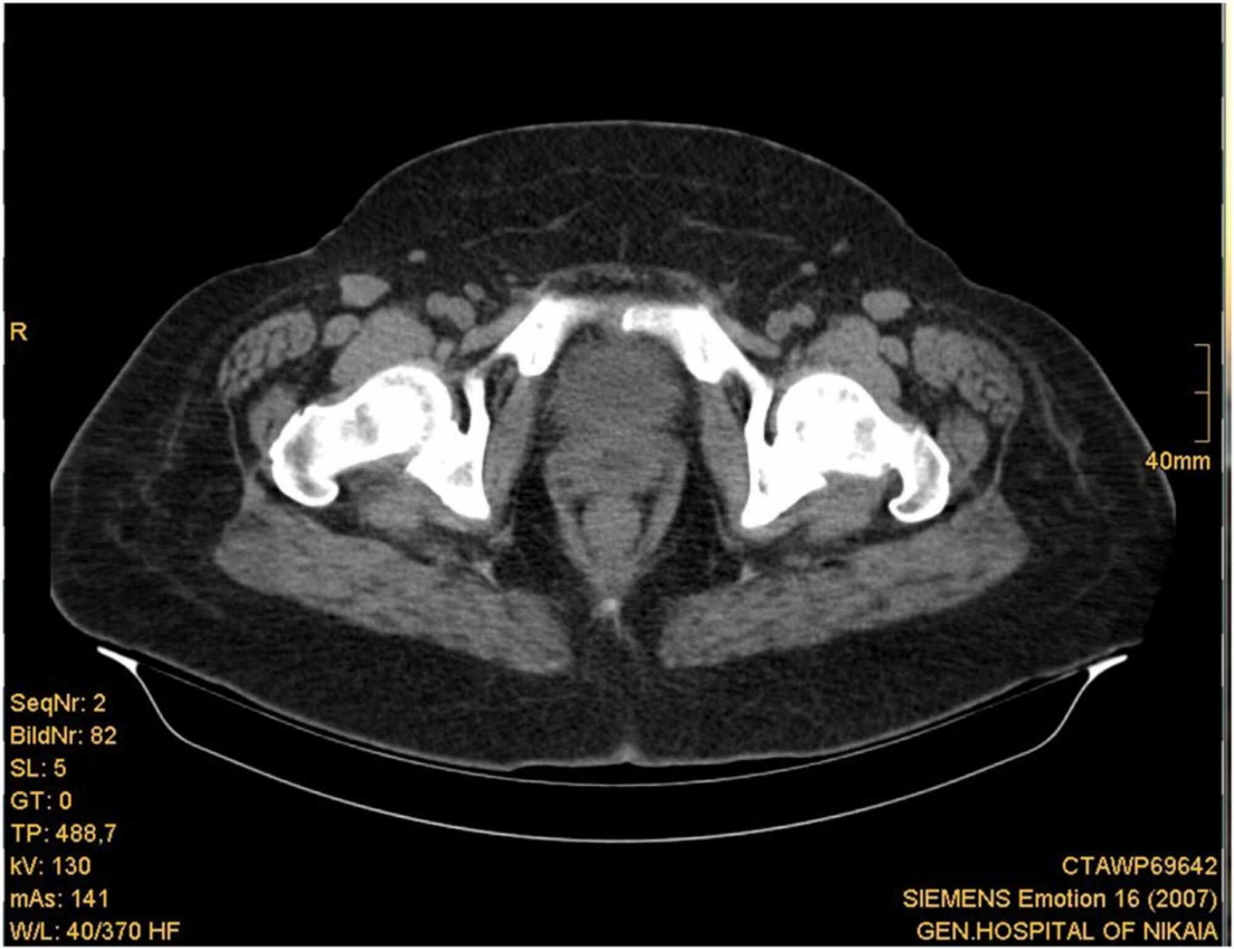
Abdominal CT scan showing the anatomic region of the uterine cervix.
